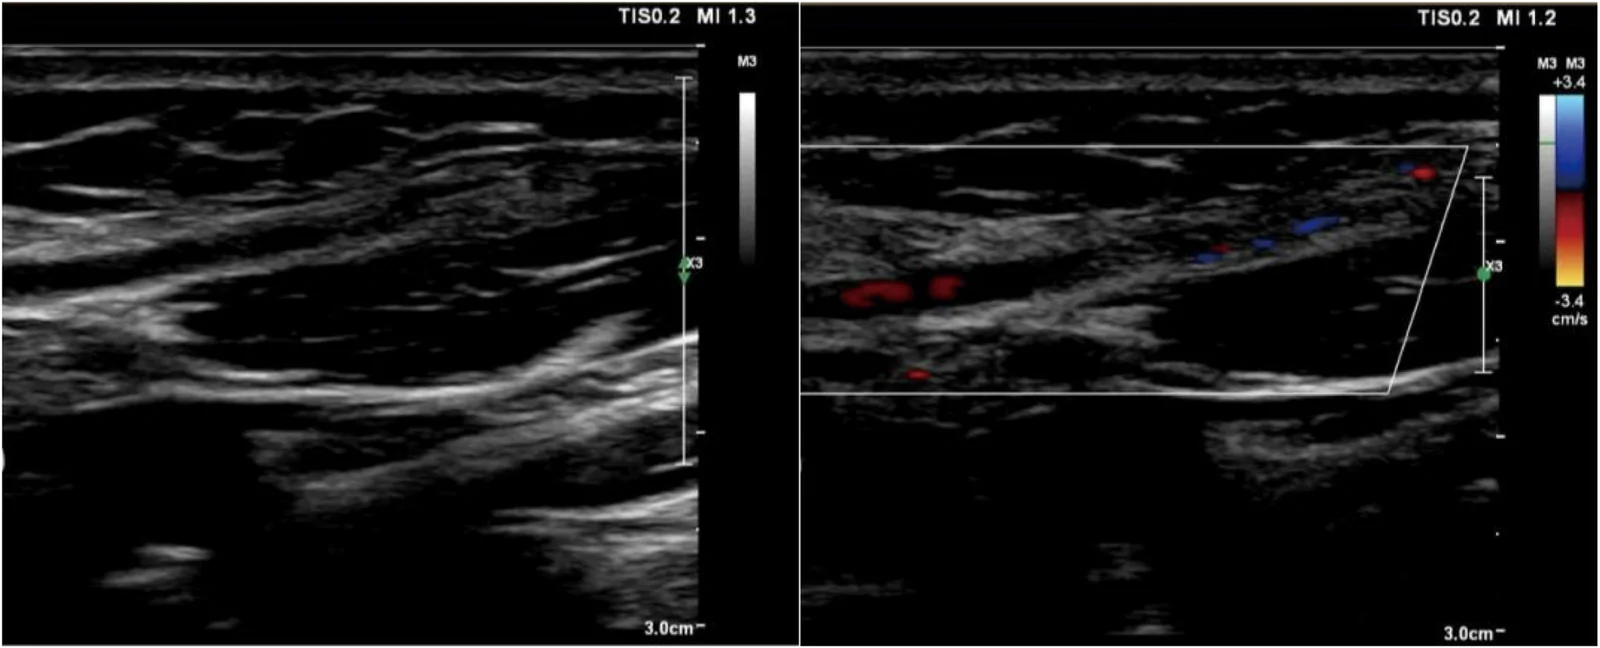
Post-operative ultrasound examination.
